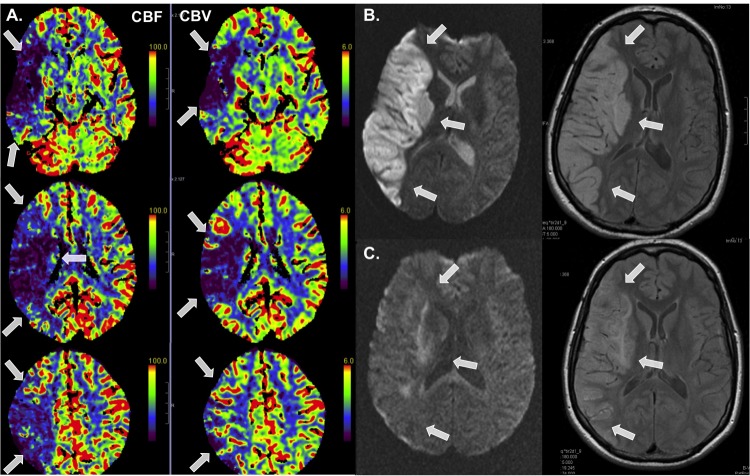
CT and MRI Scans A: Initial computed tomography (CT) perfusion (cerebral blood flow (left) and cerebral blood volume (right)) showing a large perfusion deficit in the right middle cerebral artery (MCA) area with a partial match. B and C: cranial magnetic resonance imaging (MRI, diffusion and flair weighted) two and eight days after the stroke. There is nearly no perilesional edema.
